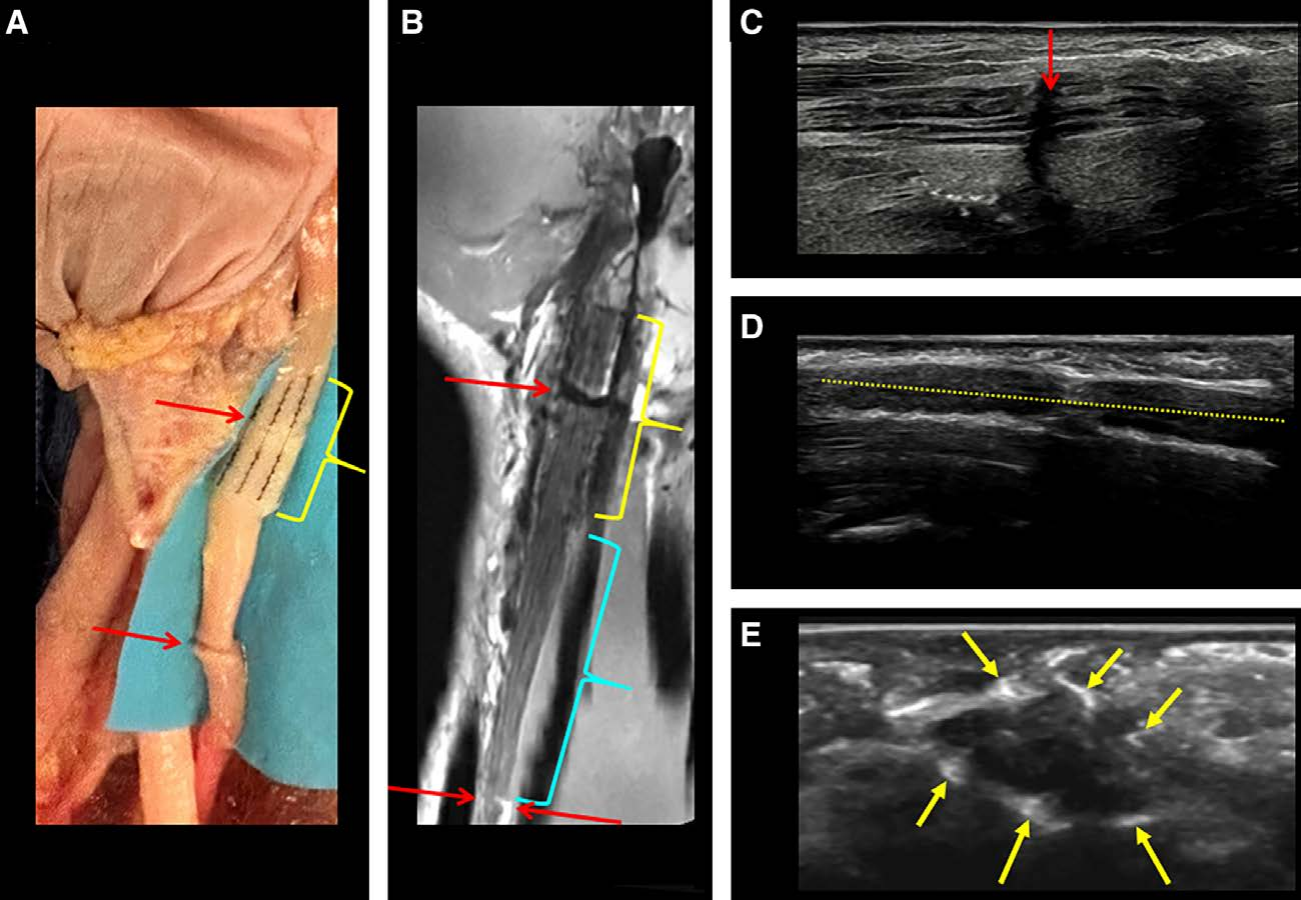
Median nerve in the cadaveric distal forearm/proximal wrist. A, Surgical implantation of NT device (NT_5_, yellow bracket) and transection sites (red arrows), with the bottom (proximal) transection repaired with sutures. B, The corresponding coronal proton density MRI demonstrates the longitudinal nerve course, NT_5_ signal void patterns (yellow bracket), and discontinuous nerve appearances at both transection sites (red arrows). The interval (L_2_) between the proximal end of NT_5_ and the proximal transections is indicated (cyan bracket). C, Longitudinal ultrasound images demonstrate both the proximal median nerve transection repaired by suture (red arrow), and (D) hyperechoic signal along the NT (yellow dotted line), whereas (E) axial ultrasound demonstrates the hyperechoic foci of the microhooks (arrows).
